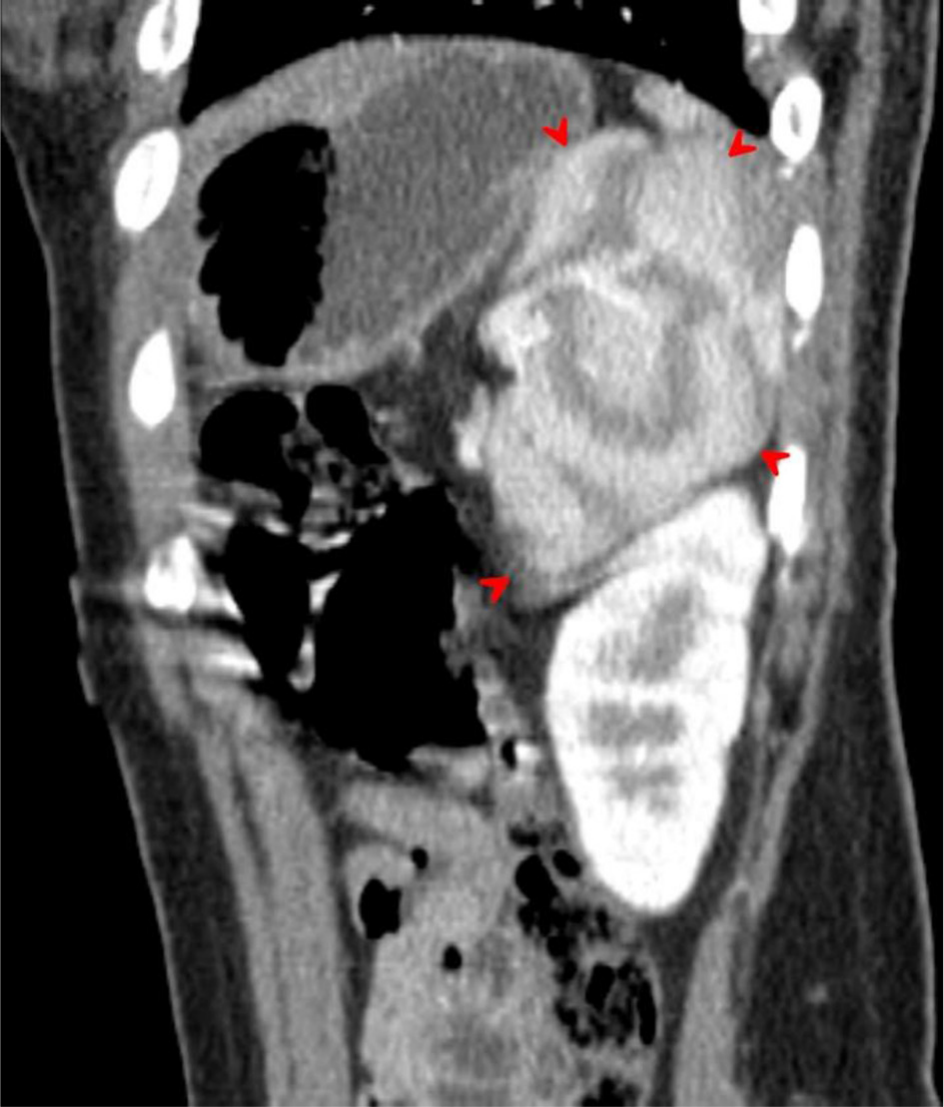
Sagittal multiplanar reformation CT image acquired during the arterial phase demonstrates the medial (internal) lobe of the spleen (red arrowheads), extending medially in a transverse manner, displacing the upper pole of left kidney downwards and gently indenting posterior aspect of the gastric fundus.
